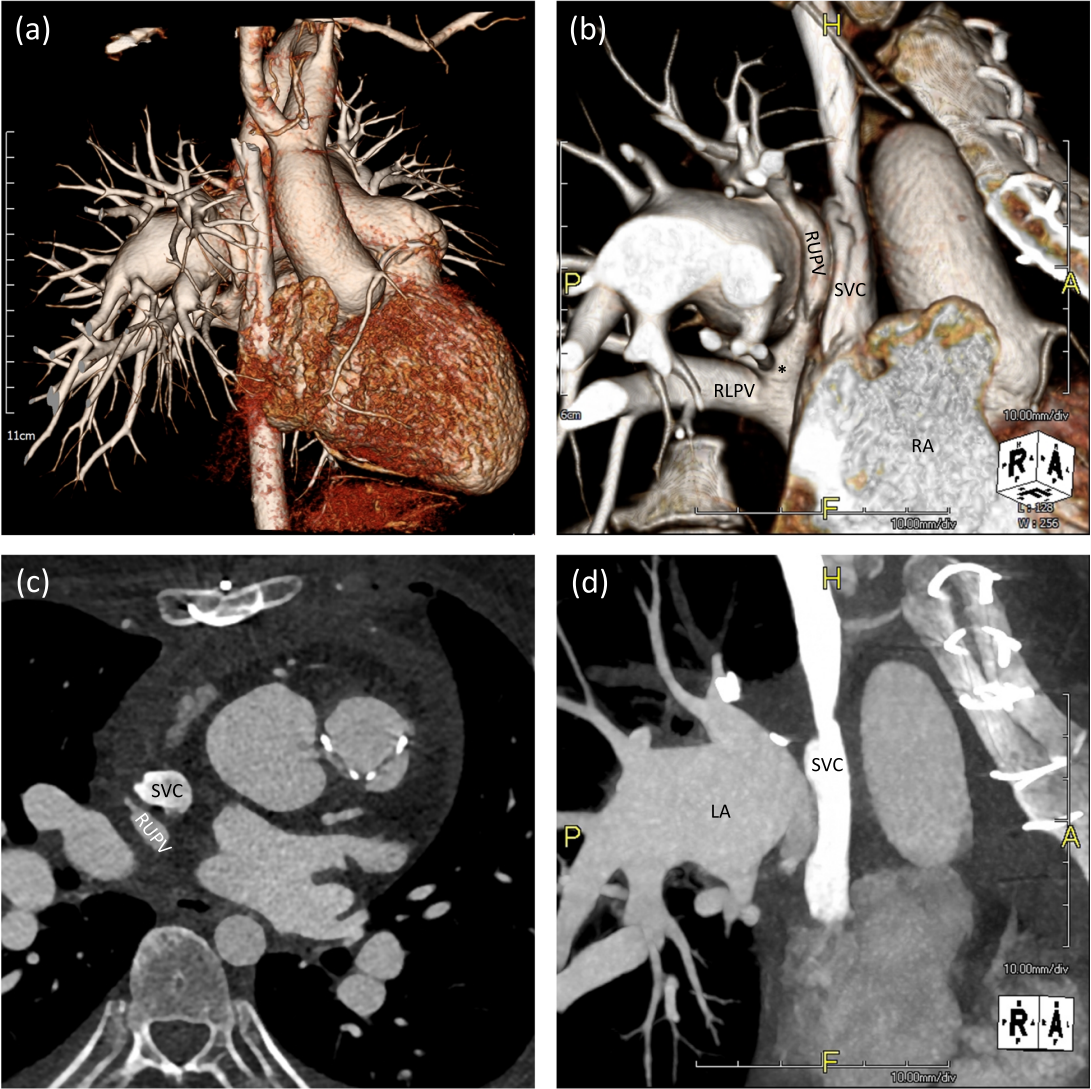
Postoperative chest computed tomography with (a) 3-dimensional reconstruction and (b) well-constructed SVC and pulmonary veins. Computed tomography demonstrating (c) RUPV and (d) LA separated from SVC. RUPV, right upper pulmonary vein; RMPV (asterisk), right middle pulmonary vein; RLPV, right lower pulmonary vein; SVC, superior vena cava; RA, right atrium; LA, left atrium
